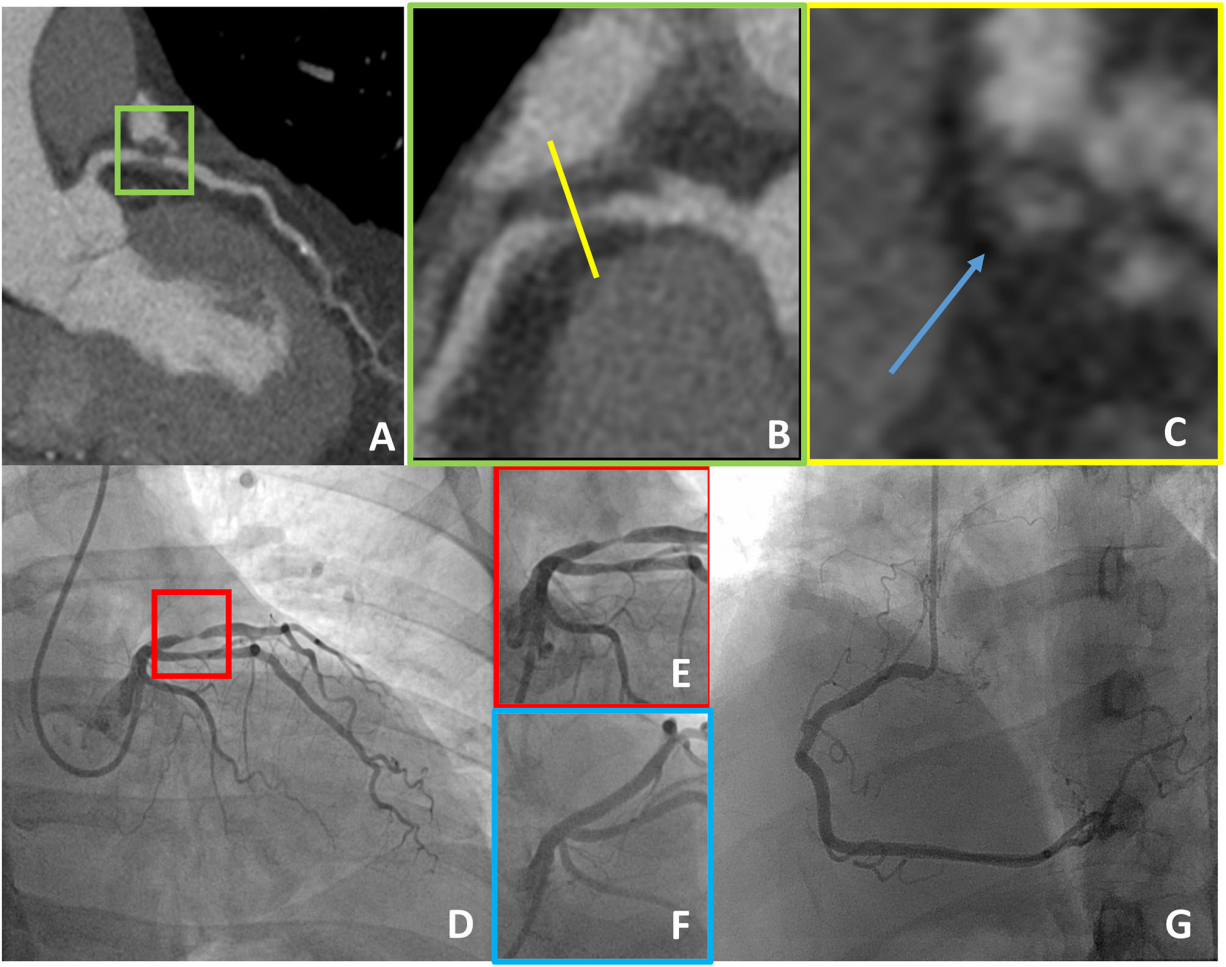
A case example of a 55-year-old man with the typical chest pain in whom CCTA detected critical stenosis of the proximal LAD (A,B), showing at the same time high-risk plaque features (positive remodeling and low-attenuation plaque as demonstrated in a short axis view, blue arrow in C). The patient underwent ICA that confirmed subocclusive disease of the proximal LAD that was treated with PCI (D–G). CCTA, coronary computed tomography angiography; LAD, left anterior descending artery; ICA, invasive coronary angiography; PCI, percutaneous coronary intervention.
